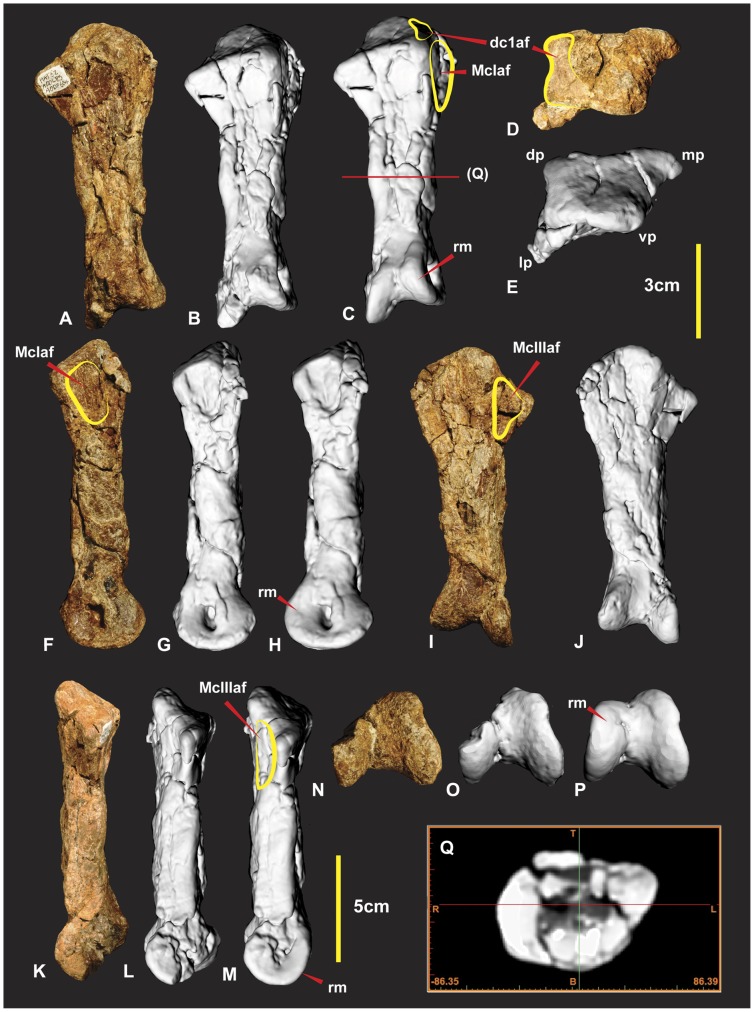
Right Metacarpal II. Right Metacarpal II in dorsal (A, B & C), proximal (D & E), medial (F, G & H), ventral (I & J), lateral (K, L & M), distal (N, O & P) views, mid shaft CT image (Q). Computer generated renders with modified clay surfaces to represent missing elements are displayed in (C, H, M & P) views. *Abbreviations:* dc1af, distal carpal 1 articular facet; dp, dorsal process; lp, lateral process; mp, medial process; McIaf, metacarpal I articular facet; rm, repaired margin; McIIIaf, metacarpal III articular facet; vp, ventral process.
